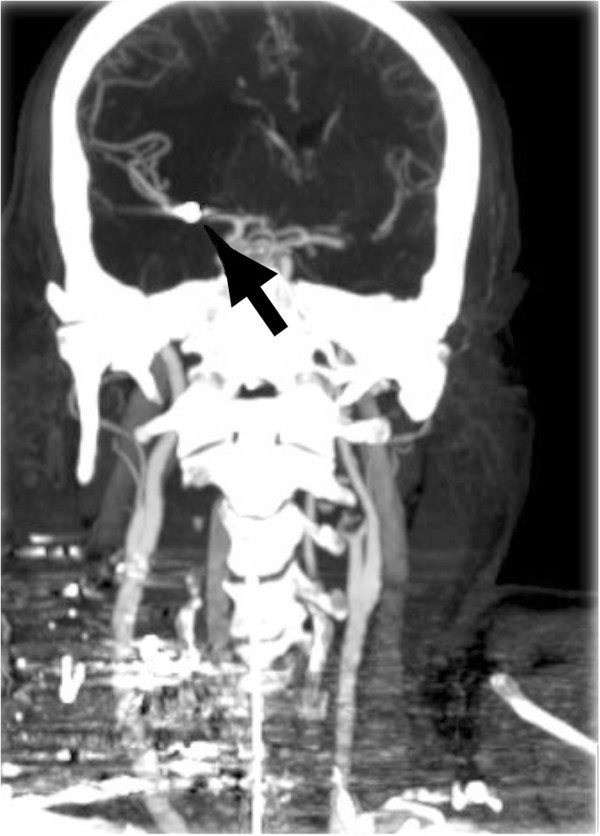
CT angiography at 24 hours post presentation.
